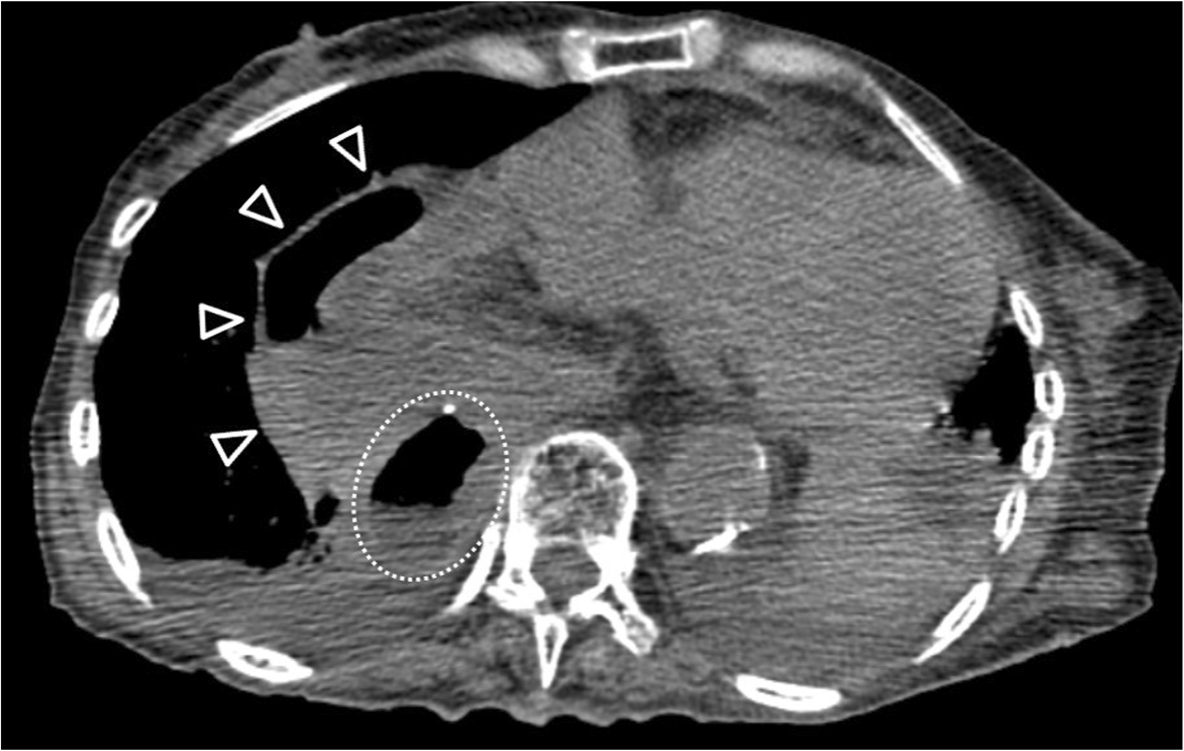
Computed tomography images. Non-contrast computed tomography revealing digestive tract prolapse into the right side of the mediastinum, which is distended with air and fluid (arrow heads). A moderate amount of pleural effusion is collected in both of the thoracic cavities. The gastric conduit is indicated in the circle
